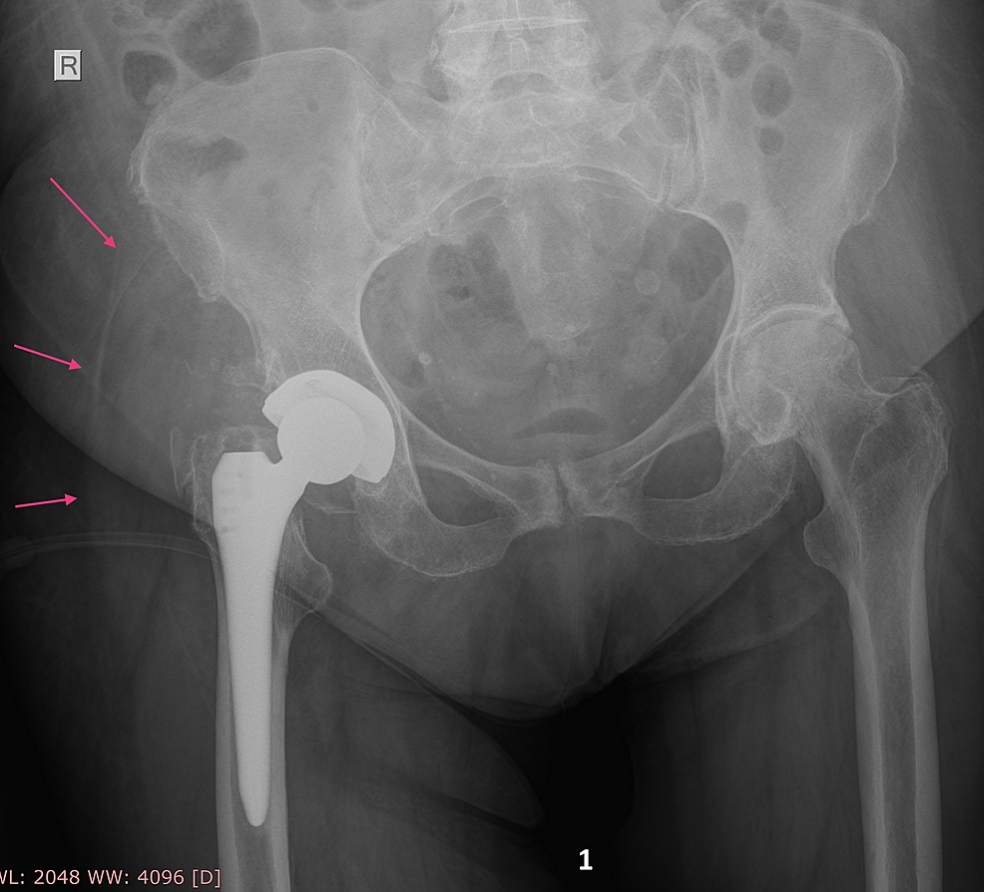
Pro-operative X-ray. Pro-operative X-ray of pelvis and right hip total arthroplasty reveals hip space effusion (red arrows) but there is no clear sign of iliopsoas abscess.
